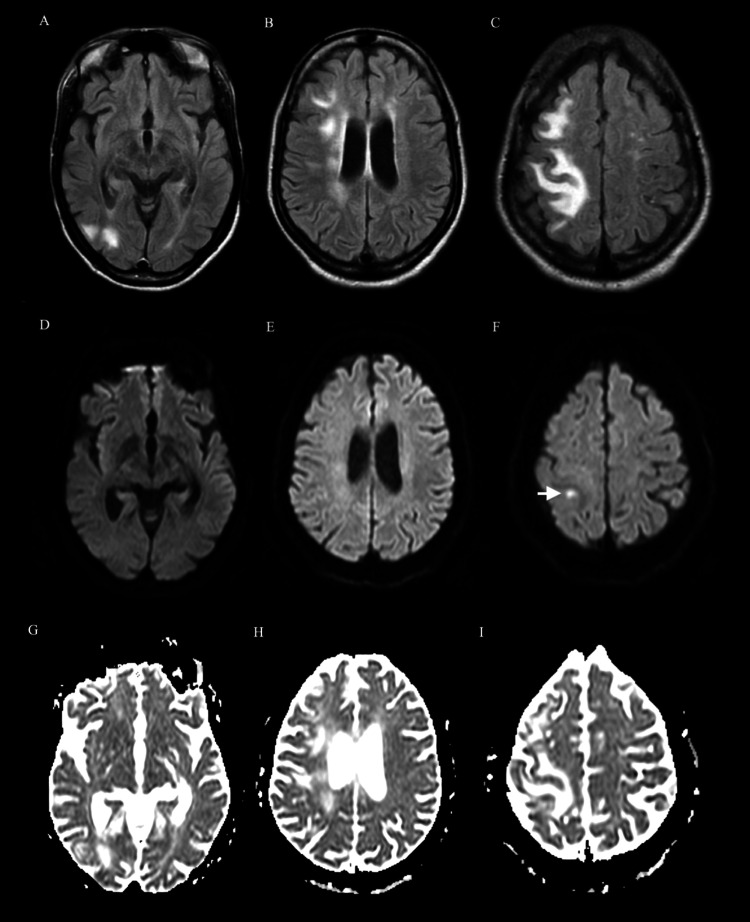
(A-I) Axial MR images performed on day three demonstrated vasogenic edema in the right cerebral hemisphere. (A-C) FLAIR sequence showing hyperintense lesions in the subcortical and deep white matter of the frontal, parietal, and occipital lobes of the right cerebral hemisphere with subtle involvement of the left hemisphere. (D-F) DWI sequence showing a tiny focus of restricted diffusion along the anterior aspect of the right post-central gyrus (white arrow). (G-I) ADC sequences showing patchy hyperintensity corresponding to the T2 shine-through effect consistent with vasogenic edema Magnetic resonance (MR); fluid-attenuated inversion recovery (FLAIR); apparent diffusion coefficient (ADC); diffusion-weighted imaging (DWI)
